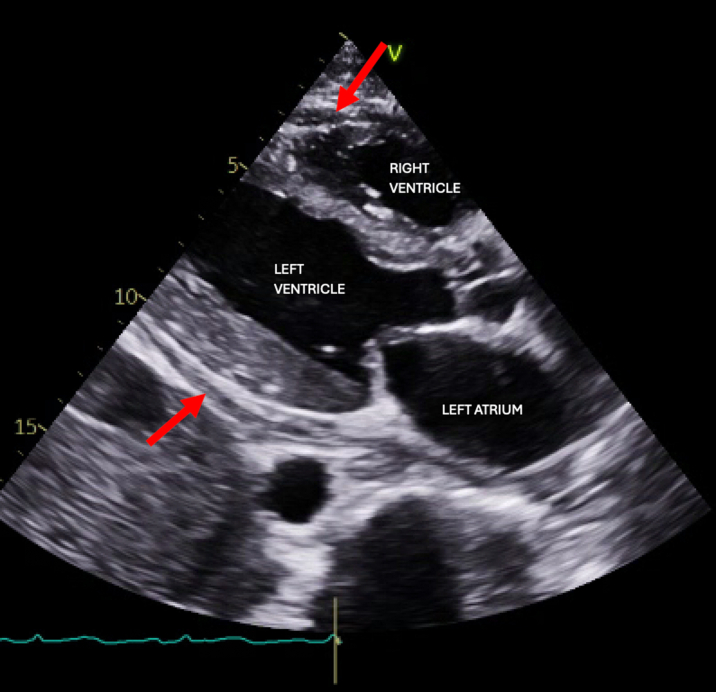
Post-treatment Echocardiogram Transthoracic echocardiogram parasternal long-axis view showing trivial, organized pericardial effusion (red arrows) 14 days after original pericardial drain placement.
